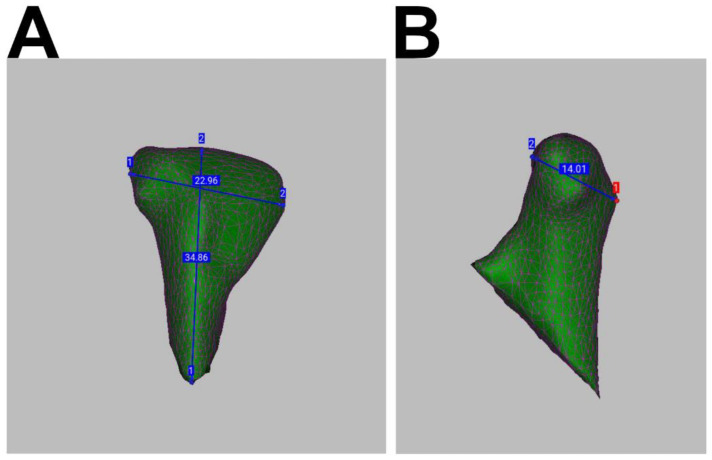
(A) Dorsal view on a follow up (T2) CBCT-scan segmentation of the left condylar neck of a 32-year-old male patient. Linear measurements at the greatest extent in transverse and longitudinal orientation are shown, performed in Artec Studio^®^. (B) Lateral view on the same segmentation as in (A) with linear measurement in the sagittal orientation.
